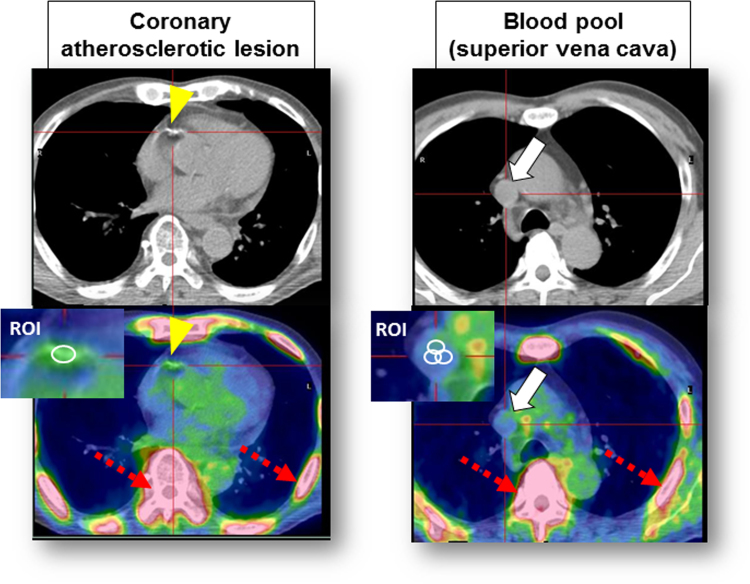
Measurements of ^18^F-NaF uptake of coronary atherosclerotic lesion (arrowheads) and blood pool (arrows) on PET/CT images. Dot-arrows indicate the intense uptake in the vertebrae and ribs.
